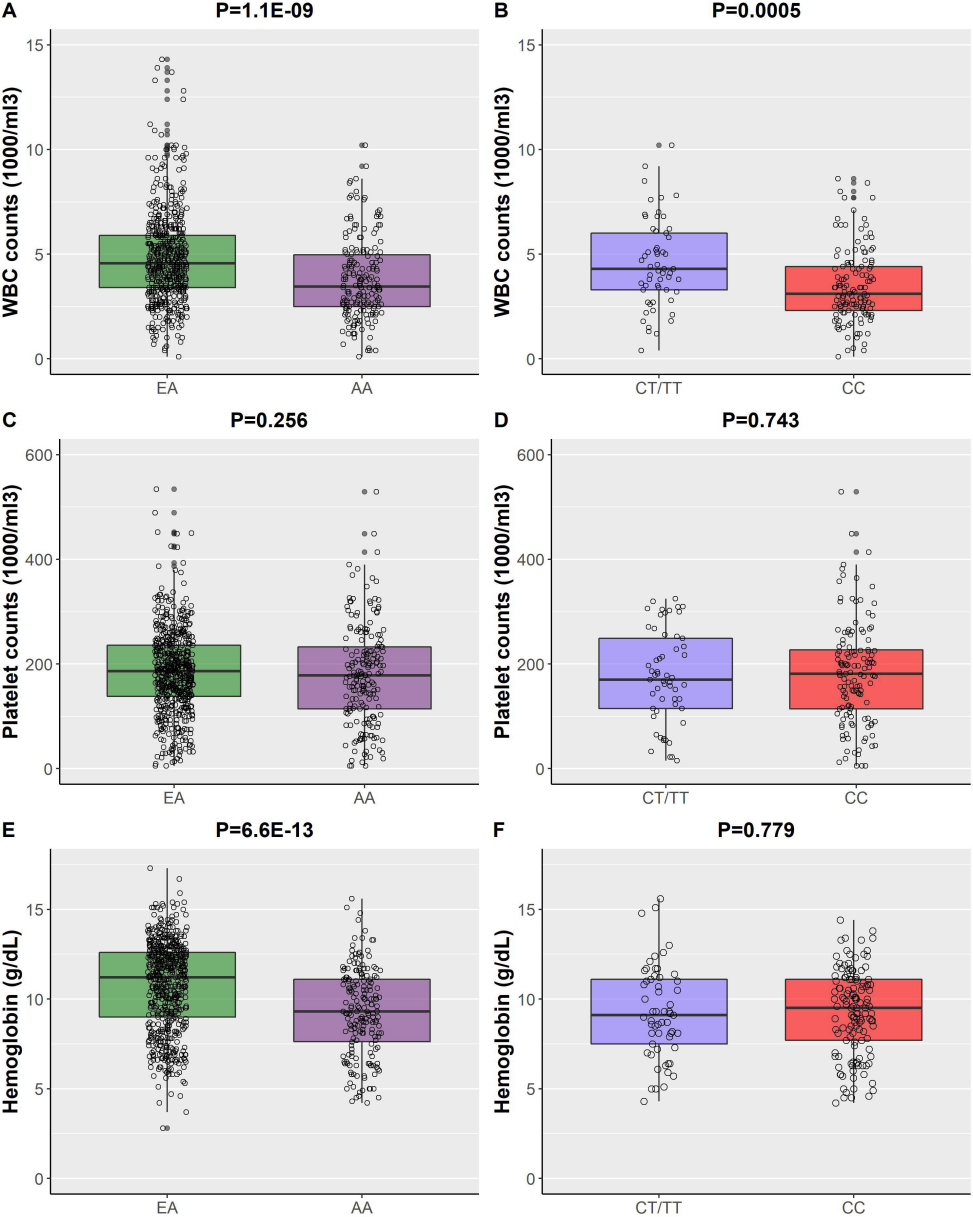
Comparisons of the lowest white blood cell (WBC) count levels (A, B), lowest platelet counts (C, D) and lowest haemoglobin levels (E, F) by ancestry and *ACKR1* genotype. Figures on the right show the comparisons between European ancestry (EA) and African ancestry (AA). Figures on the left show the comparisons between rs2814778-CT/TT and CC genotype in patients with SLE of AA.
